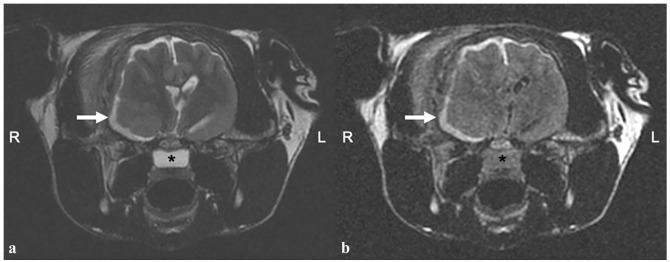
(a) Transverse T2-weighted (T2W), and (b) T2W fluid-attenuated inversion recovery (FLAIR) images of the brain. There is an ill-defined, broad-based space-occupying lesion (arrows) centred at the level of the right piriform lobe that extends along the calvarium. The mass is iso- to faintly hyperintense on T2W and T2W FLAIR images and surrounded by an irregular and focally discontinuous marked T2W and FLAIR hyperintensity that expands along the meninges. The nasopharynx is fluid filled (*)
